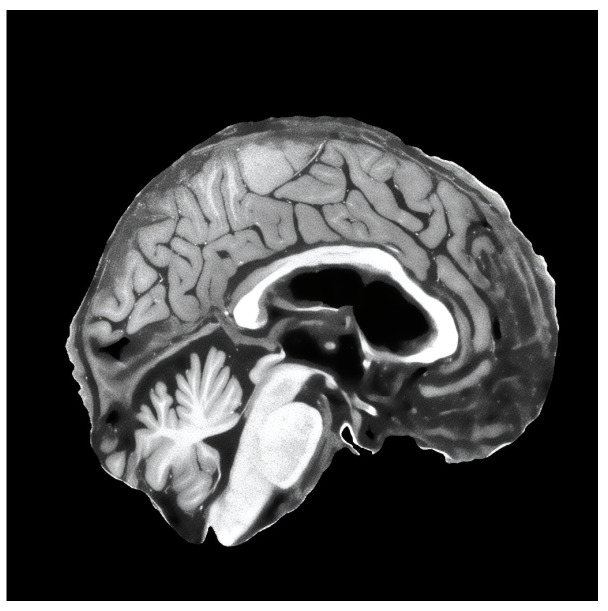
Example of a generated brain image of sagittal plane from ADNI [50] dataset, which contains black spots. The slice of the brain in this figure is taken from MPRAGE T1w MRI, which was taken with 3T MR scanner.
